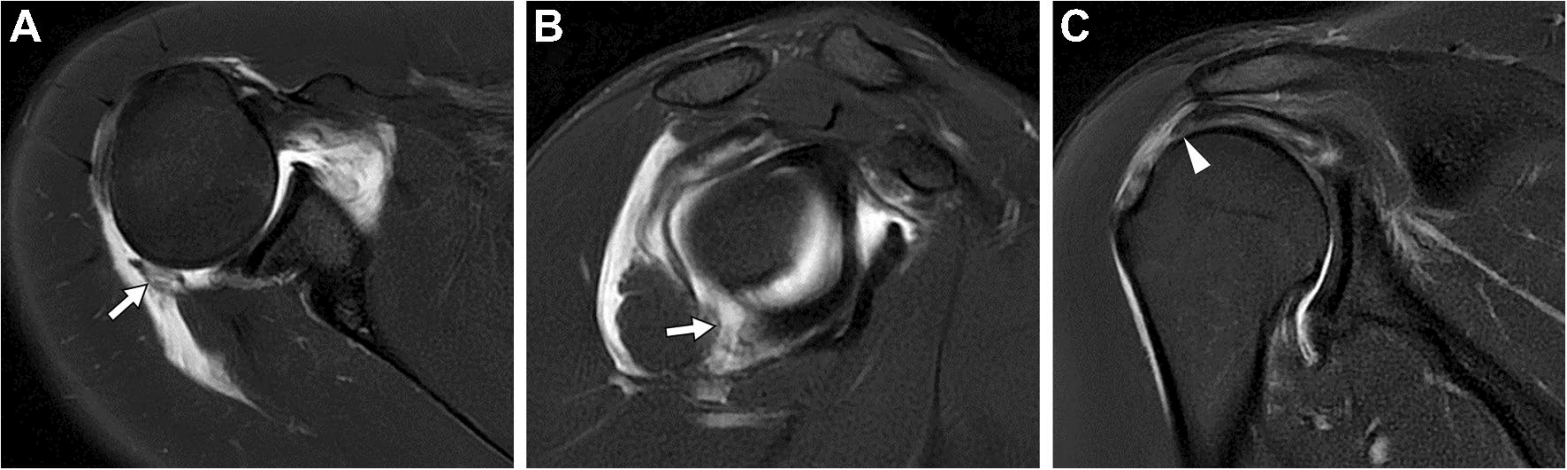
Arthrographic effect from a post-traumatic hemarthrosis/effusion. Twenty-five-year-old man who fell while playing football. Axial (A), sagittal (B), and coronal (C) T2-weighted fat-suppressed conventional 3 T MR images show a tear of the inferior glenohumeral ligament complex involving the posterior band and capsule (arrows) as well as traumatic rupture of the superior cuff (arrowhead)
